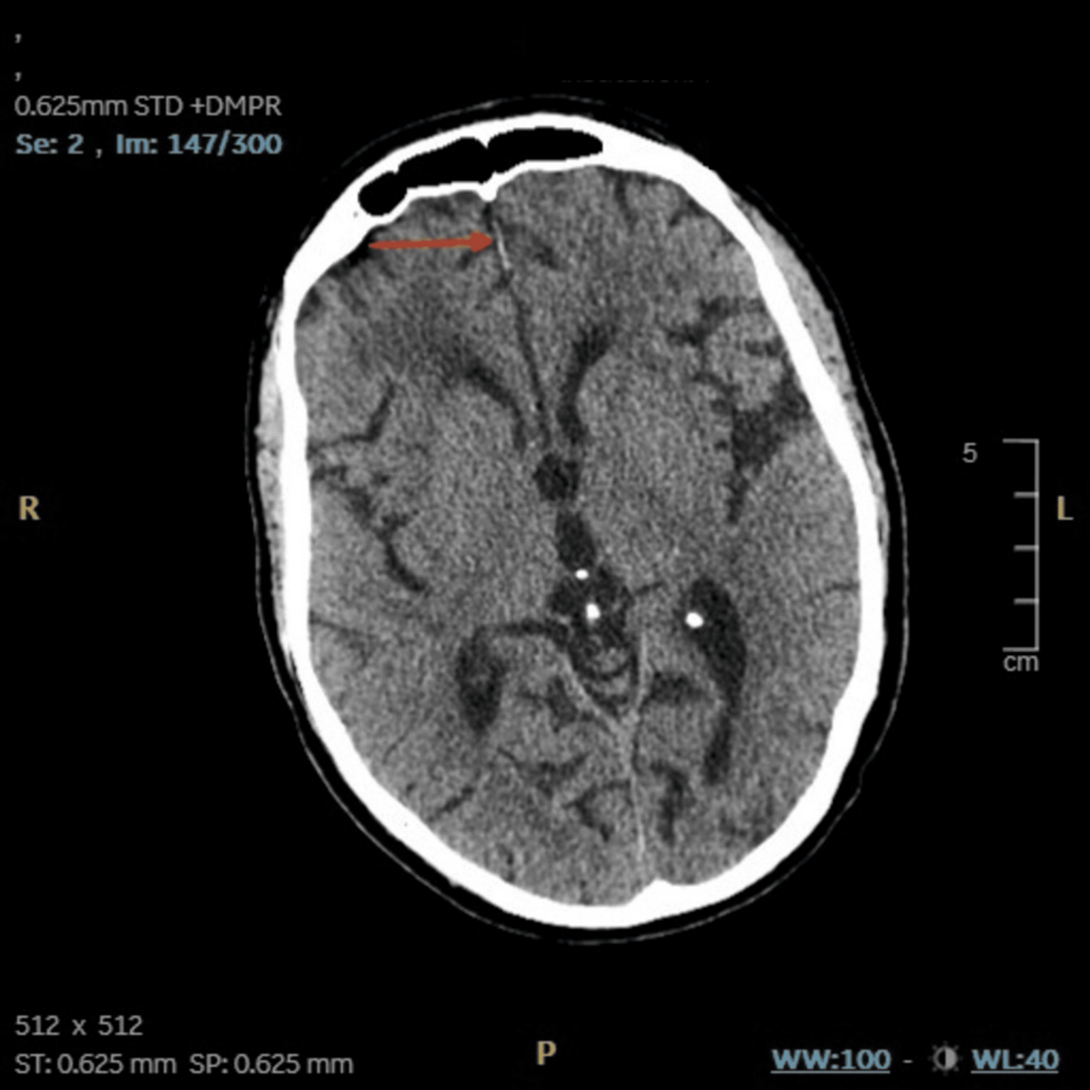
CT head - image 2 The red arrow signifies fine specks of calcification in the right corona radiata and right high frontal region CT: computed tomography
